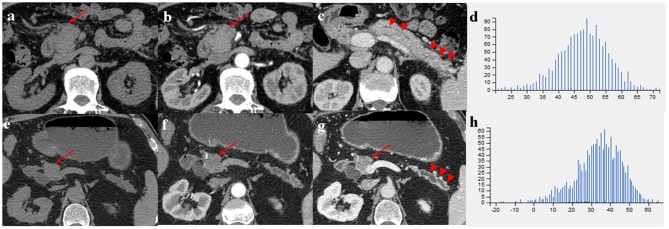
(a–c) A 66-year-old man with MFP, CT imaging showed a pancreatic head tumor (arrows) with slight duct dilatation and slight tail enlargement (arrowheads); (e–g) A 83-year-old man with PDAC, CT imaging showed a pancreatic head tumor (arrows) with severe duct dilatation and obvious tail atrophy (arrowheads). (d,h) Histograms of texture parameters of the two lesions showed a marked difference.
